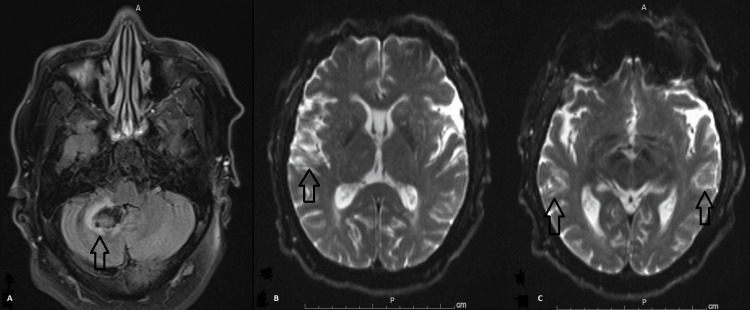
Initial MRI brain Magnetic resonance imaging (MRI) brain with and without contrast showing right-sided cerebella hemorrhagic stroke (arrow in A), bilateral cerebral hemisphere acute/sub-acute infarctions mainly affecting watershed areas, centrum semiovale, and corona radiata (arrows in B and C)
